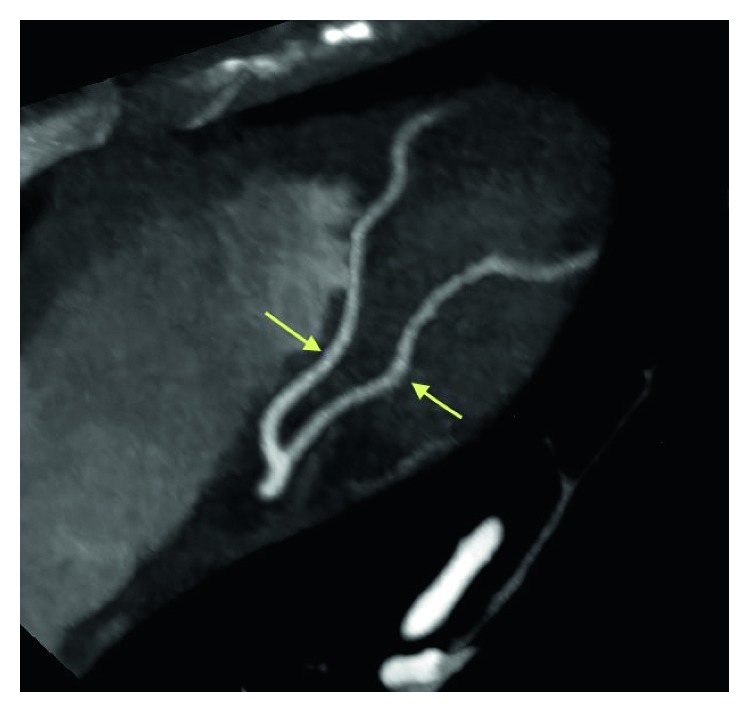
Coronary CT angiogram image revealing the distal segments and diagonal branches of LAD are normal without any evidence of coronary artery disease.
